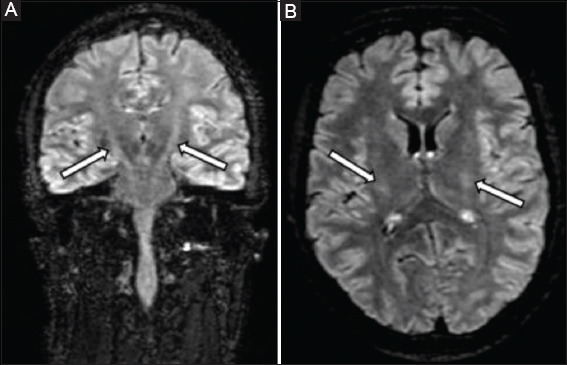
Magnetic resonance imaging cerebrum, at 4 months of follow-up. (A) T2. (B) Fluid-attenuated inversion recovery: Bilateral hyperintensities of the corticospinal tract (white arrows).
